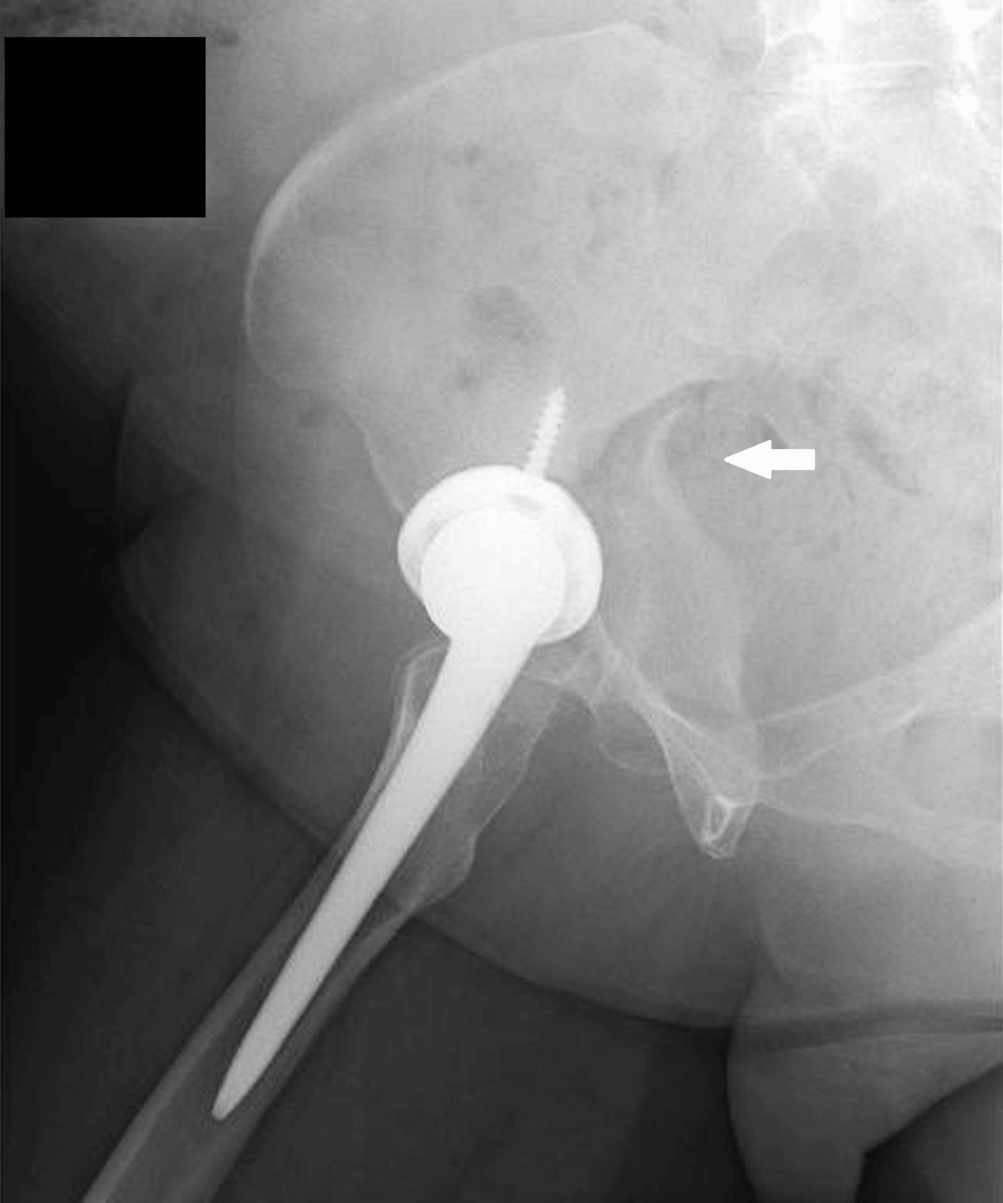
Plain radiograph of the pelvis at initial presentation (lateral view). A right acetabular fracture was observed (arrow). There was no clear evidence of implant loosening.
